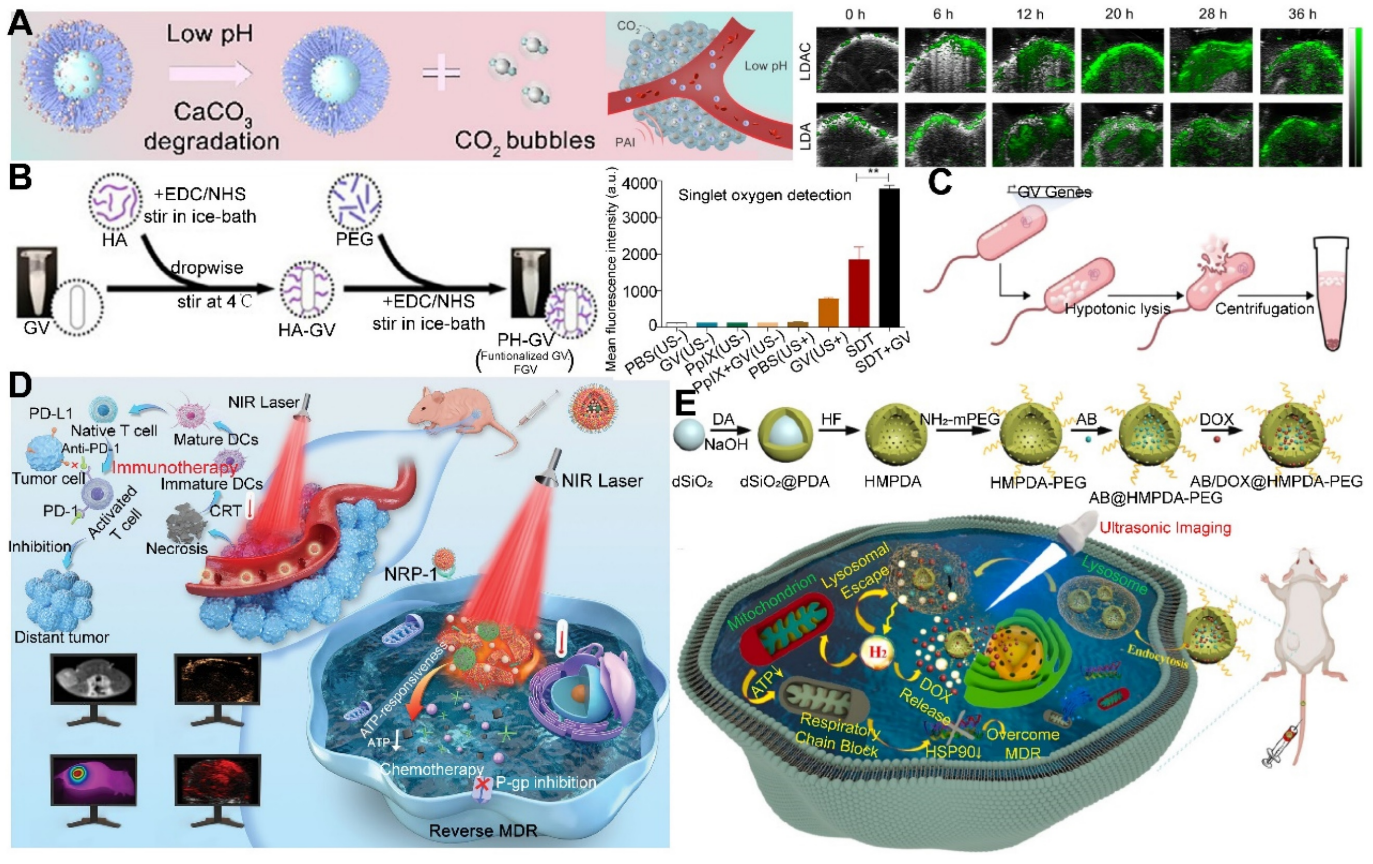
Application of gas therapy in cancer imaging. (A) A nanoplatform (LDAC NPs) used for PAI/ NIR-II FI dual-mode imaging. Adapted with permission from 155, copyright 2023 Elsevier. (B) A nanoplatform designed to achieve SDT and ultrasonic imaging (GVs). Reproduced with permission from 159, copyright 2021 Elsevier. (C) Improved GVs with stable ultrasonic contrast signal. Reproduced with permission from 160, copyright 2022 Mingjie Wei *et al.* (D) A nanoplatform designed for ultrasonography imaging, PAI, and MRI (P@TF NPs). Reproduced with permission from 164, copyright 2023 Wiley-VCH. (E) A nanoplatform AB/DOX@HMPDA-PEG for improving ultrasonic imaging efficacy. Reproduced with permission from 166, copyright 2021 Elsevier.
